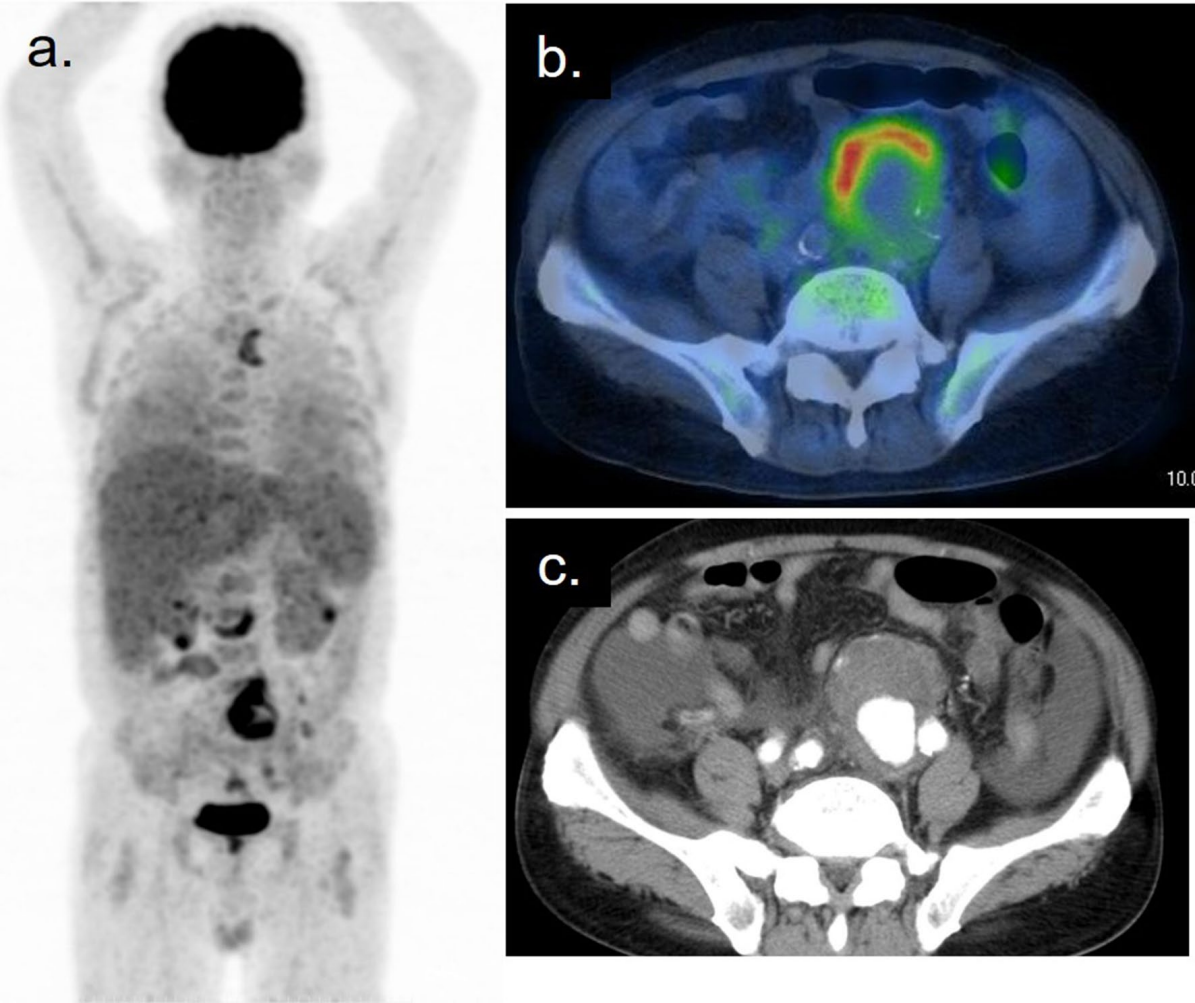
Ectopic granuloma at the abdominal aortic wall and disseminated granuloma in the lungs, liver, bone marrow, kidneys are visualized on the FDG–PET (a) [23]. The patient has a history of transurethral resection of bladder tumor and 8 times intravesical BCG injection for his bladder cancer. The axial PET/CT fusion image (b) shows intense accumulation on wall of the dilated left common iliac artery. Five days after the PET/CT examination, this patient developed an acute abdomen with impending rupture of this aneurysm (c)
